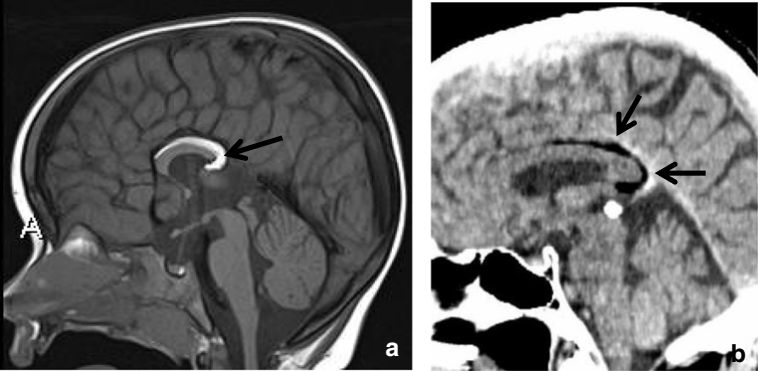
Two-year-old child. Sagittal T1 shows dysgenesis of the corpus callosum mostly affecting the splenium, with a curvilinear lipoma extending from below the splenium continuing anteriorly, covering the superior surface of the corpus up to the genu (a). Seventy-two-year-old man. Sagittal CT reconstruction shows a sharply demarcated hypodense structure curving posteriorly around a normal appearing splenium, consistent with a lipoma (black arrows). The bright spot inferior to the lipoma is a calcified pineal gland (b)
